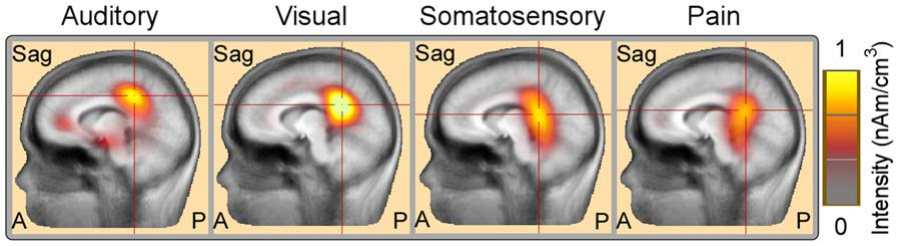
Source localizations of P300 elicited by target stimuli across all sensory modalities. Distributed sources estimated around P300 peak latencies using CLARA are superimposed on standard MR image template, and the color is coded according to their intensity, expressed in nAm/cm^3^. Talairach coordinates (x, y, z) of the sources of P300 are -9, -41, 37 mm; -9, -47, 19 mm; -9, -41, 13 mm; and -9, -47, 25 mm for auditory, visual, somatosensory, and pain target conditions respectively. Note that the sources of the P300 elicited by target stimuli across all sensory modalities are similarly located at the posterior cingulate cortex.
